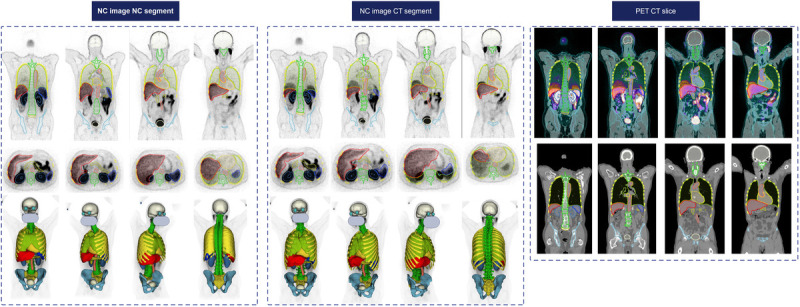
^68^Ga-PSMA PET/CT image with respiratory mismatch. It should be noted that the kidney boundaries are expanded in the visualized images due to selected window width/level. Face is masked for privacy.
